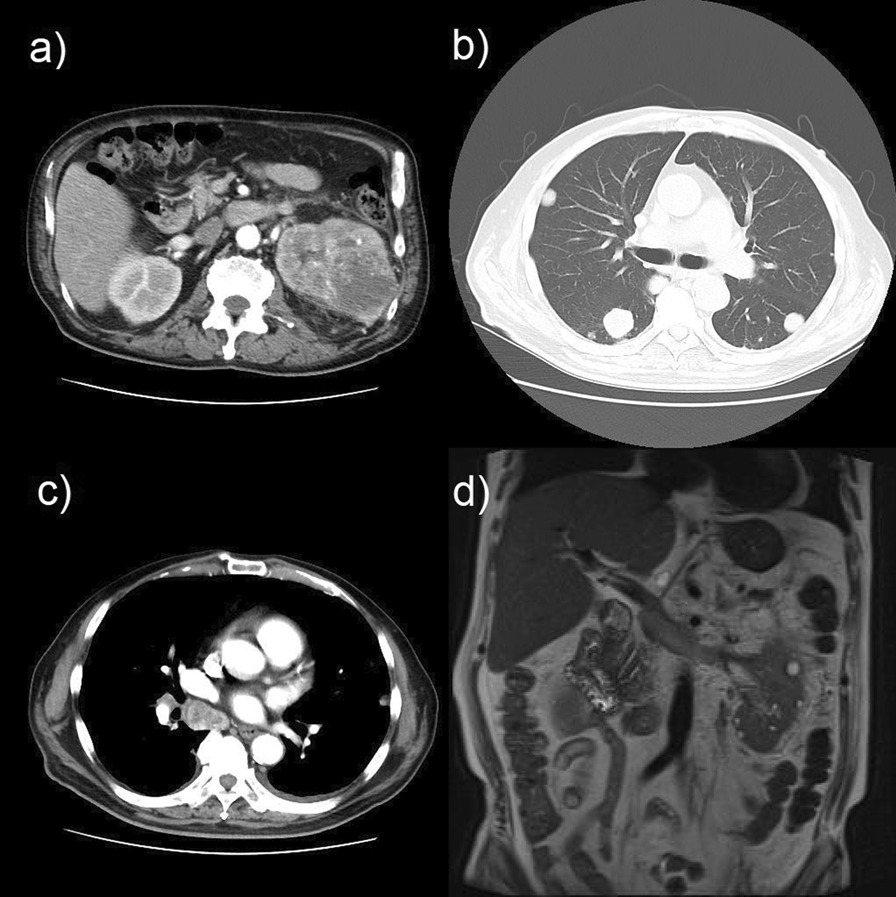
Primary and metastatic lesion suggestive of renal cell carcinoma at the first visit. Computed tomography shows a left renal mass (a), multiple lung masses (b), and mediastinal lymph node enlargement (c). Magnetic resonance imaging shows a level II tumor thrombus reaching the inferior vena cava (d)
